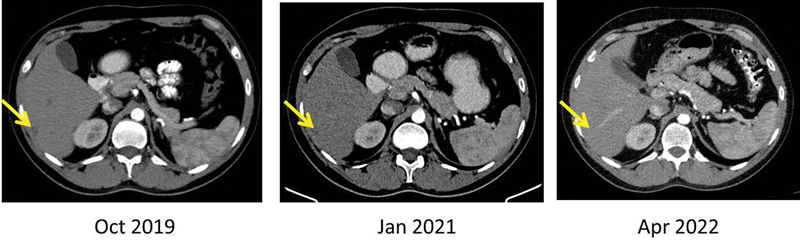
Computed tomography (CT) images were acquired in the last few visits, which showed that the liver lesion was stable. Here, Arrows show the near total resolution of the metastatic liver lesion.
